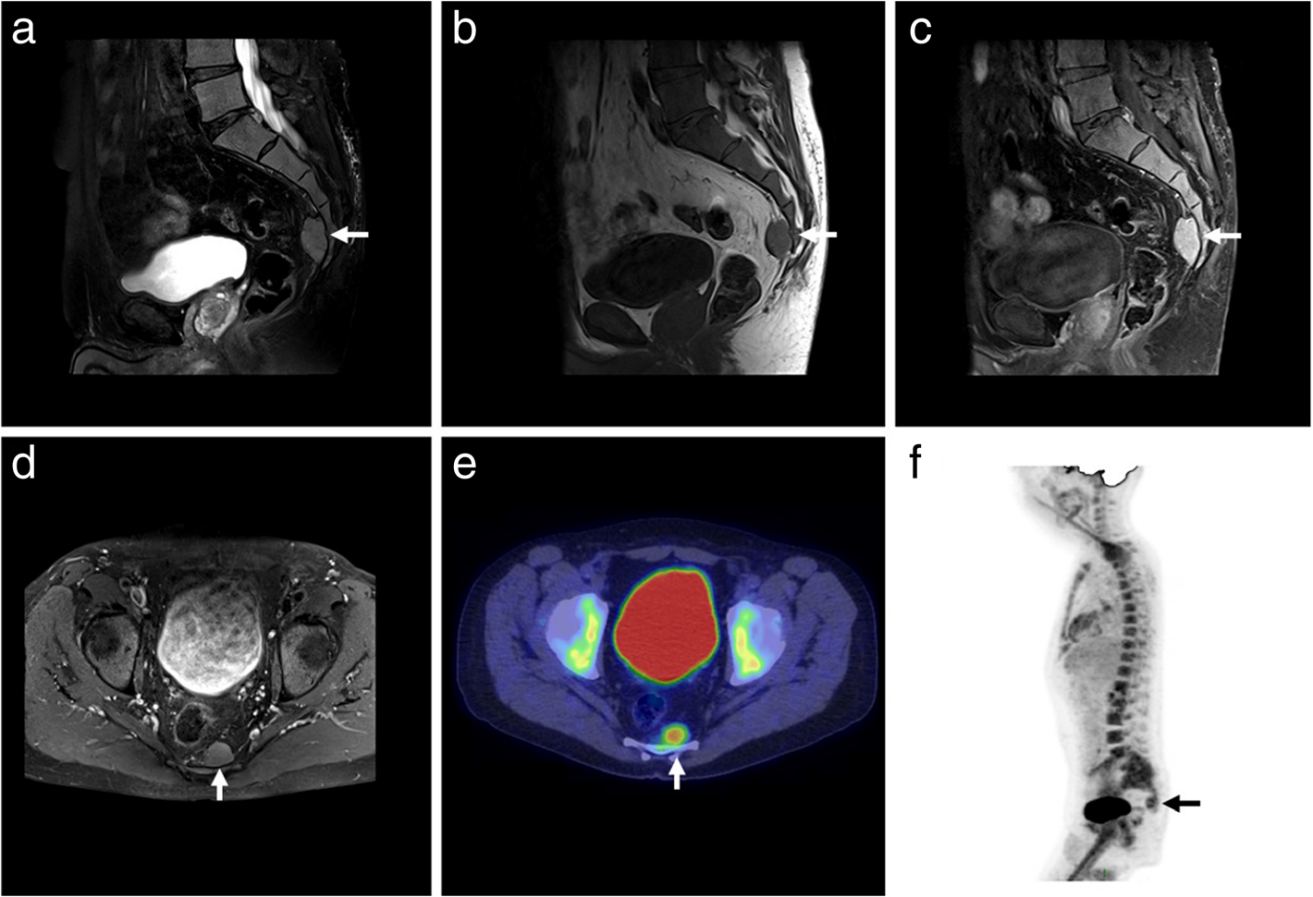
Presacral extramedullary haematopoiesis. a Sagittal. b T2. c Pre-contrast T1. d Post-contrast T1FS. e Axial post-contrast T1FS. f Fusion PET/CT with colour map. FDG-18 PET/CT MIP reconstruction demonstrating a presacral lesion with increased FDG uptake, consistent with presacral extramedullary haematopoiesis. Note the ‘superscan’ appearance from diffuse FDG uptake in the axial and appendicular skeleton (f)
